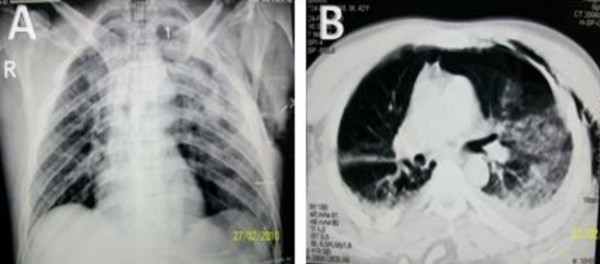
Anteroposterior chest X-ray and CT scan chest demonstrating the predictability of occult pneumothorax. AP chest X-ray revealing evidence of bilateral lung contusions and left subcutaneous emphesema (Panel A). Chest CT confirming both the lung contusions and the subcutaneous emphesema and demonstrating left sided pneumothorax not initially appearing on the anteroposterior chest X-ray (Panel B). Adapted from Omar et. al.[6].
